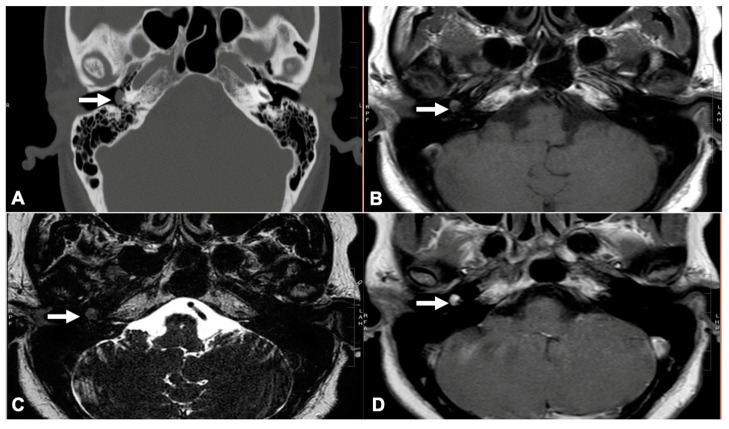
(A) A glomus tympanicum (white arrow) appears as a homogeneous soft tissue mass located at the cochlear promontory surface, as observed on the axial HRCT; (B) The glomus tympanicum (white arrow) in non-enhanced T1-weighted MRI; (C) The glomus tympanicum (white arrow) in T2-weighted MRI; (D) The glomus tympanicum (white arrow) limited in the middle ear cleft in contrast-enhanced T1-weighted MRI. HRCT, high-resolution computed tomography; MRI, magnetic resonance imaging.
